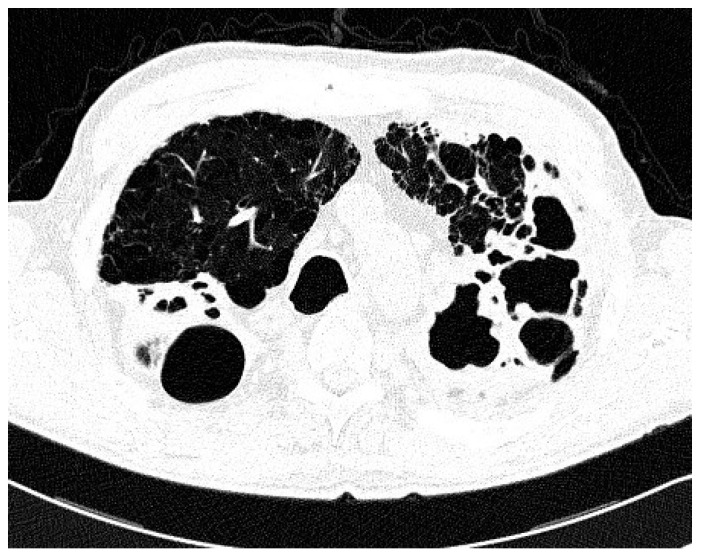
Trans-axial lung window CT scan in patient with non-tuberculous mycobacteria lung disease (NTM-LD) and chronic obstructive pulmonary disease (COPD) shows emphysema and multiple thick-walled cavities in both upper lobes.
